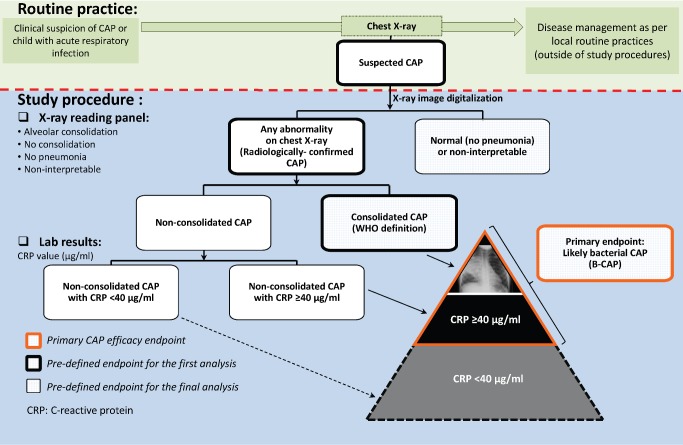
Chest X-ray classification and CAP end point definitions.
